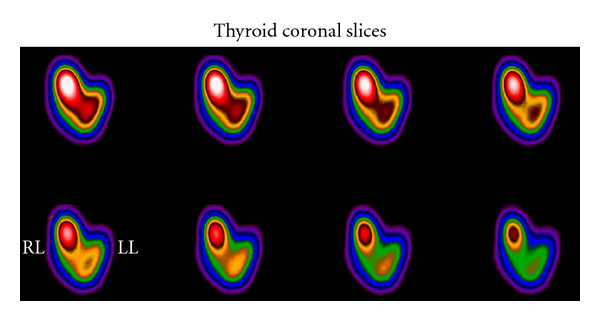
Sequential coronal slices of Thyroid SPECT study. Reconstruction made by FBP and Ramp-Butterworth prefiltering. Right lobe node delineation. *Study is presented courtesy of M. Gavrilelli, MSc, “Medical Imaging Center” Athens, Greece. *
